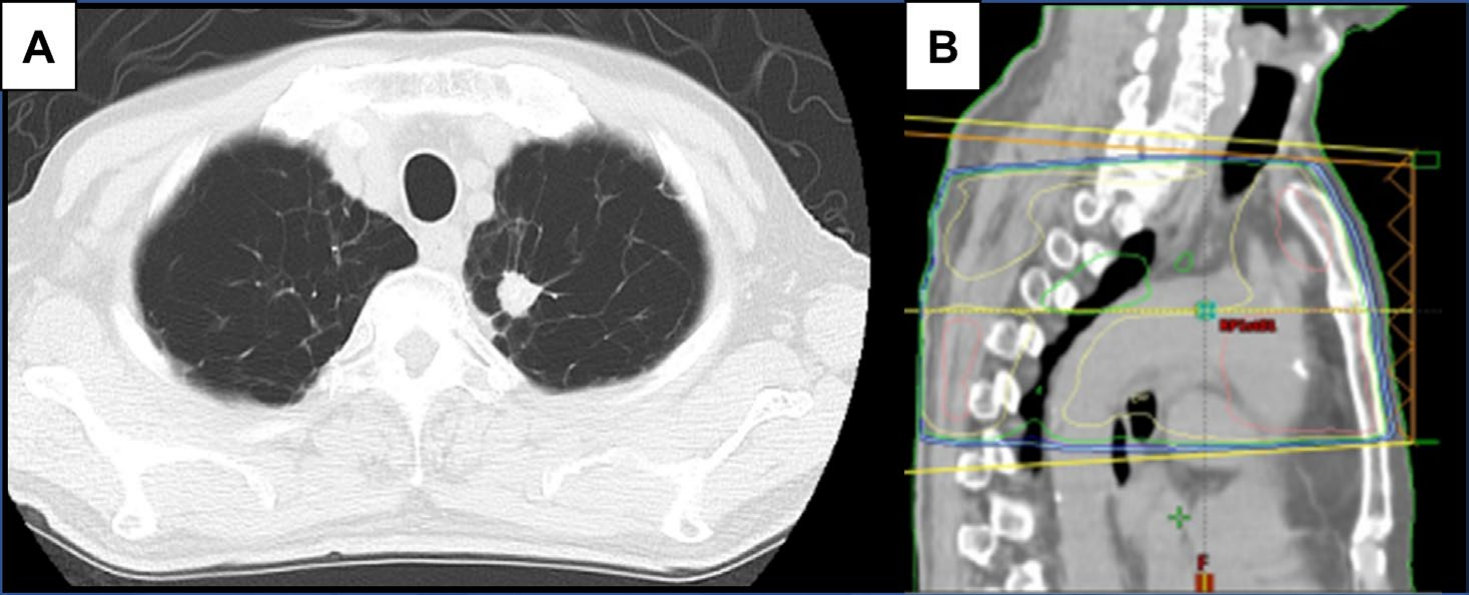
(A) Chest CT shows emphysematous changes and a 2.5 cm mass in the left upper lobe. (B) Intensity‐modulated radiation therapy was administered at a total dose of 60 Gy in 30 fractions over 6 weeks, targeting the primary tumor and ipsilateral mediastinal lymph nodes.
